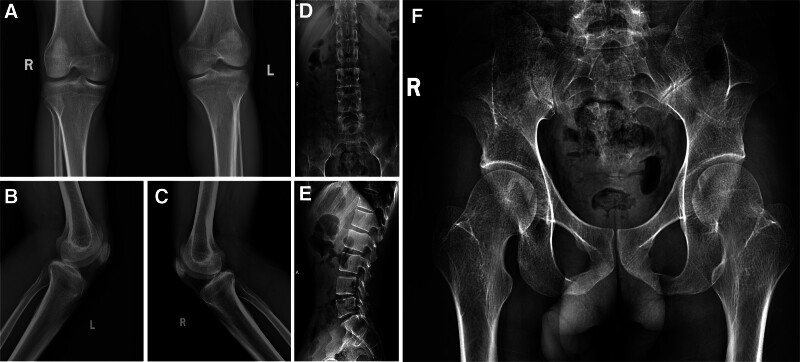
(A–C) AP and lateral knee radiographs. (D) AP lumbar spine radiograph. (E) Lateral lumbar. (F) AP pelvis radiograph. AP = anteroposterior.
